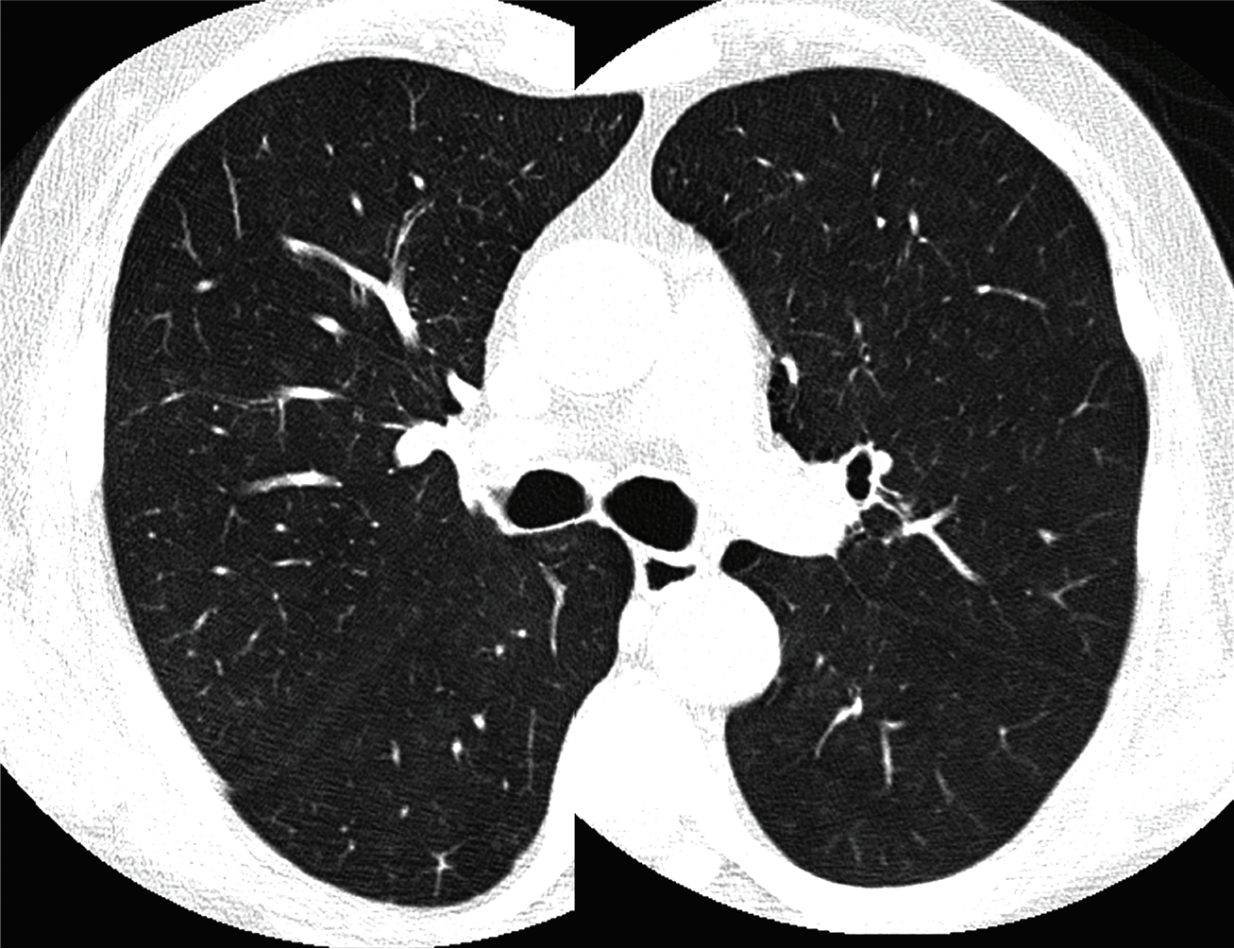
High-resolution computed tomography image. No structural abnormality is seen in the lung field by high-resolution computed tomography.
